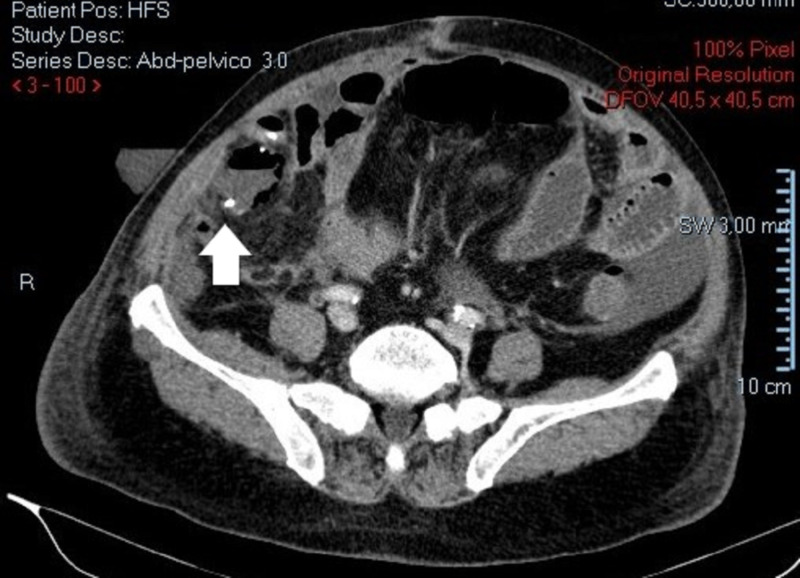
Contrast enhanced abdominopelvic CT scan. Free air around previous site of colonic anastomosis consistent with anastomotic dehiscence.
